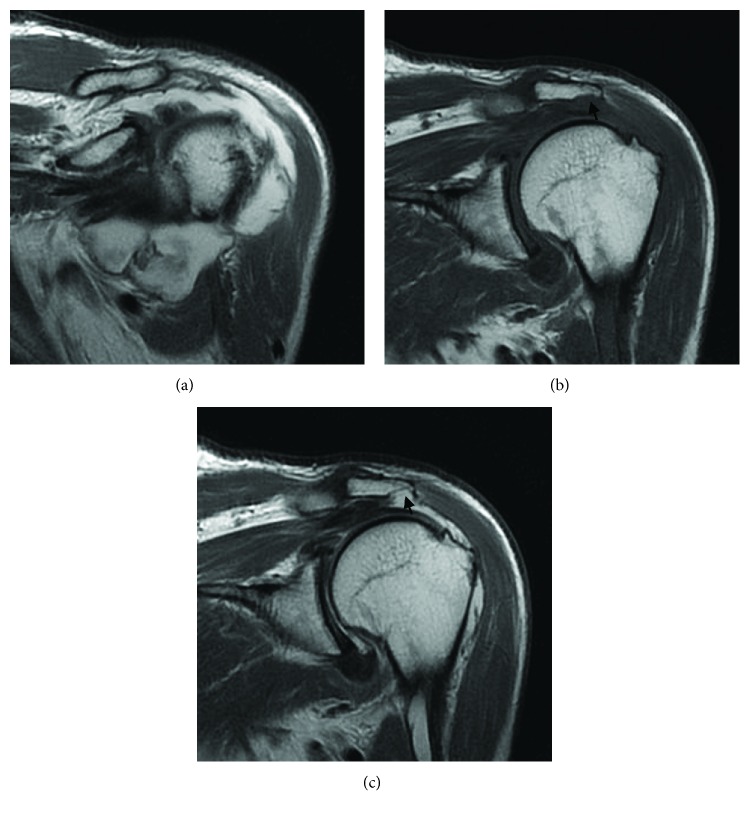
Oblique coronal magnetic resonance images of the left shoulder. A hematoma was seen in the anterior portion of the subacromial bursa in T2-weighted images (a). Subacromial bony erosion was detected in T1- (b) and T2-weighted images (c) (arrows). Bursal-side partial tear was also seen.
